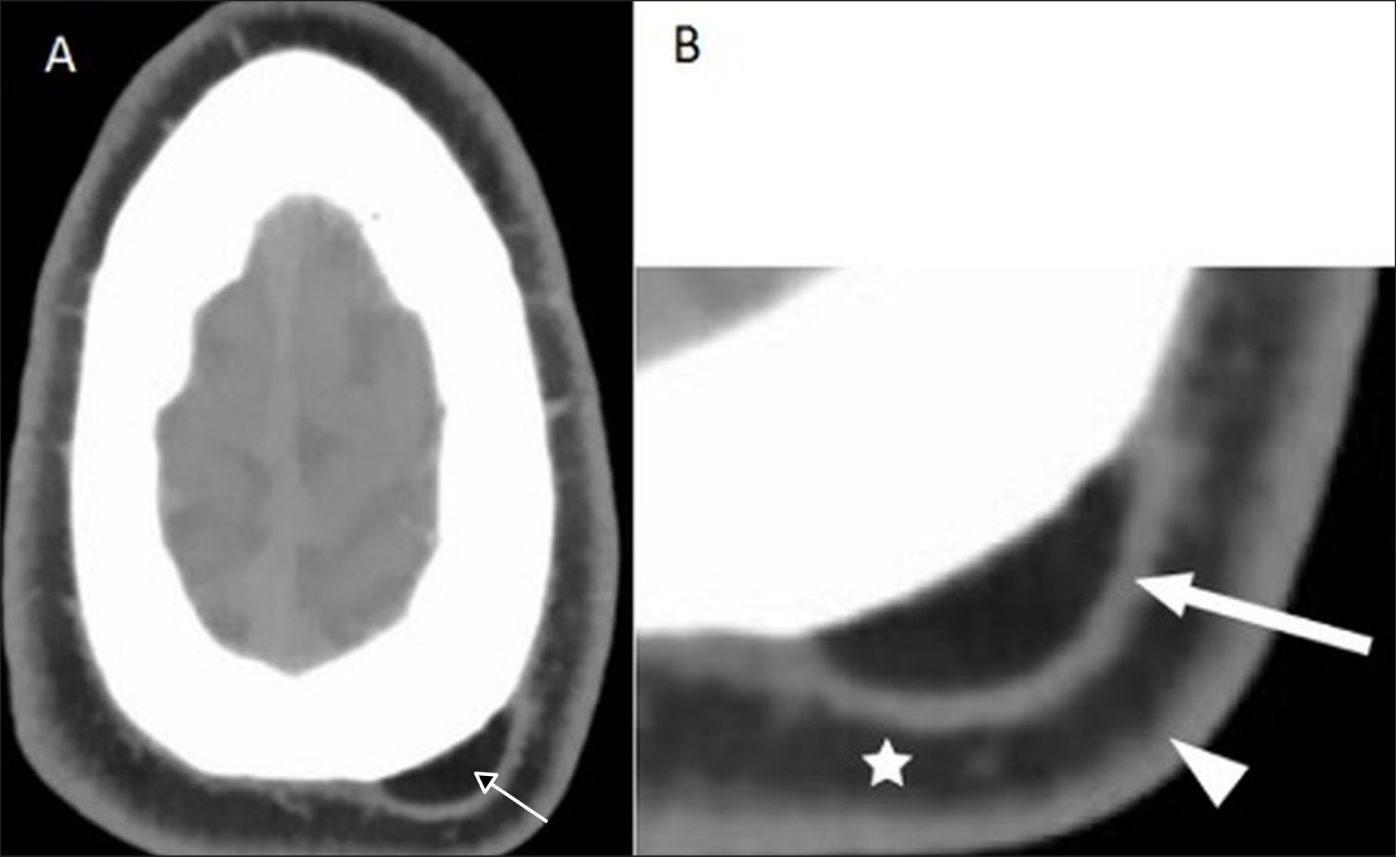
Unenhanced CT scan (axial view). (A) A fat-containing mass located under the galea aponeurosis. (B) An enlarged image showing the different layers of the scalp distinguishable on CT imaging: skin (white arrowhead), subcutaneous fat (white star), galea aponeurosis (white arrow).
